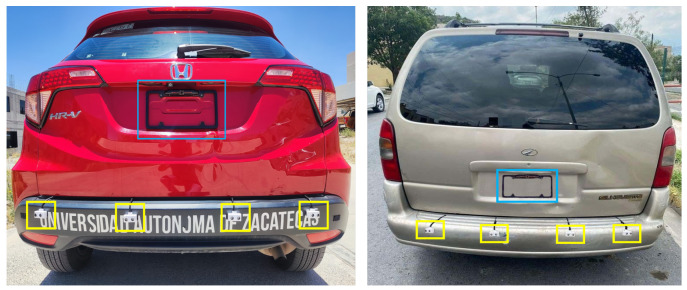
Experimental setup. The blue box = reverse camera installed, yellow boxes = ultrasonic sensors.
